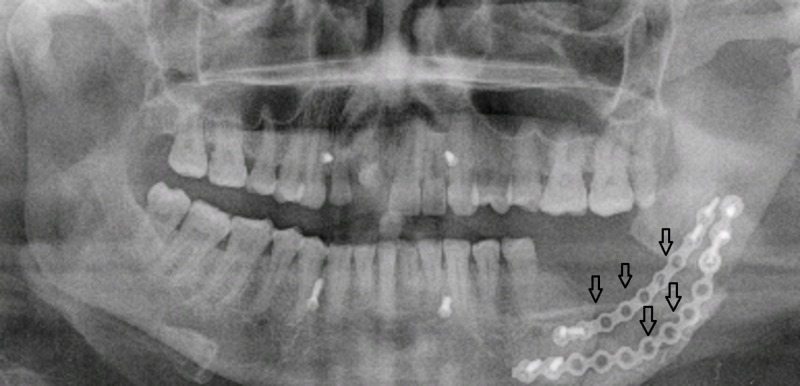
Patient 4. A 55-year-old male with left-sided facial swelling. Postoperative orthopantomogram shows reconstruction of mandible with plating (arrows).
